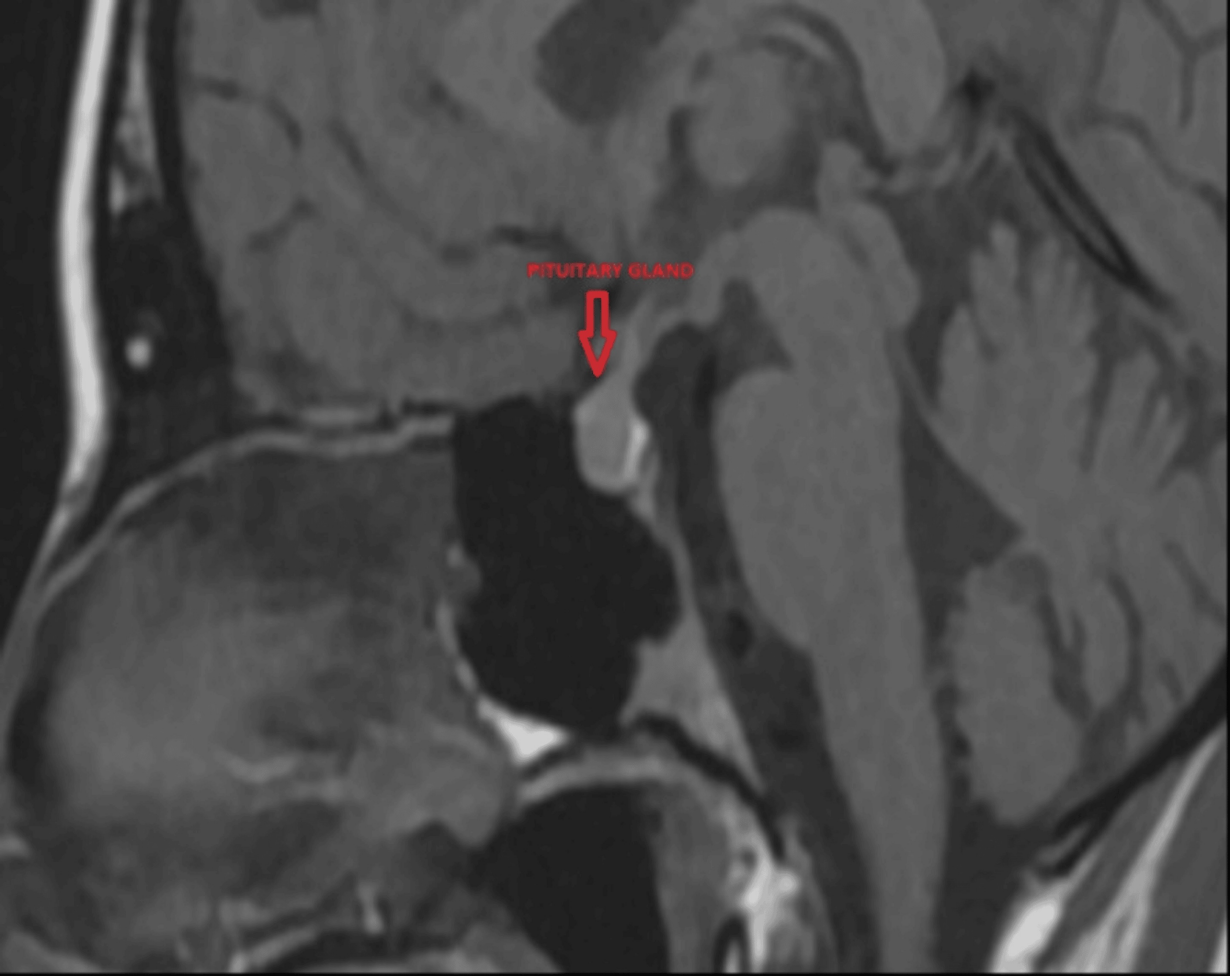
MRI of the pituitary gland - native series, sagittal plane Pituitary microadenoma type "dark" with discrete compression of the infundibulum. MRI: magnetic resonance imaging
